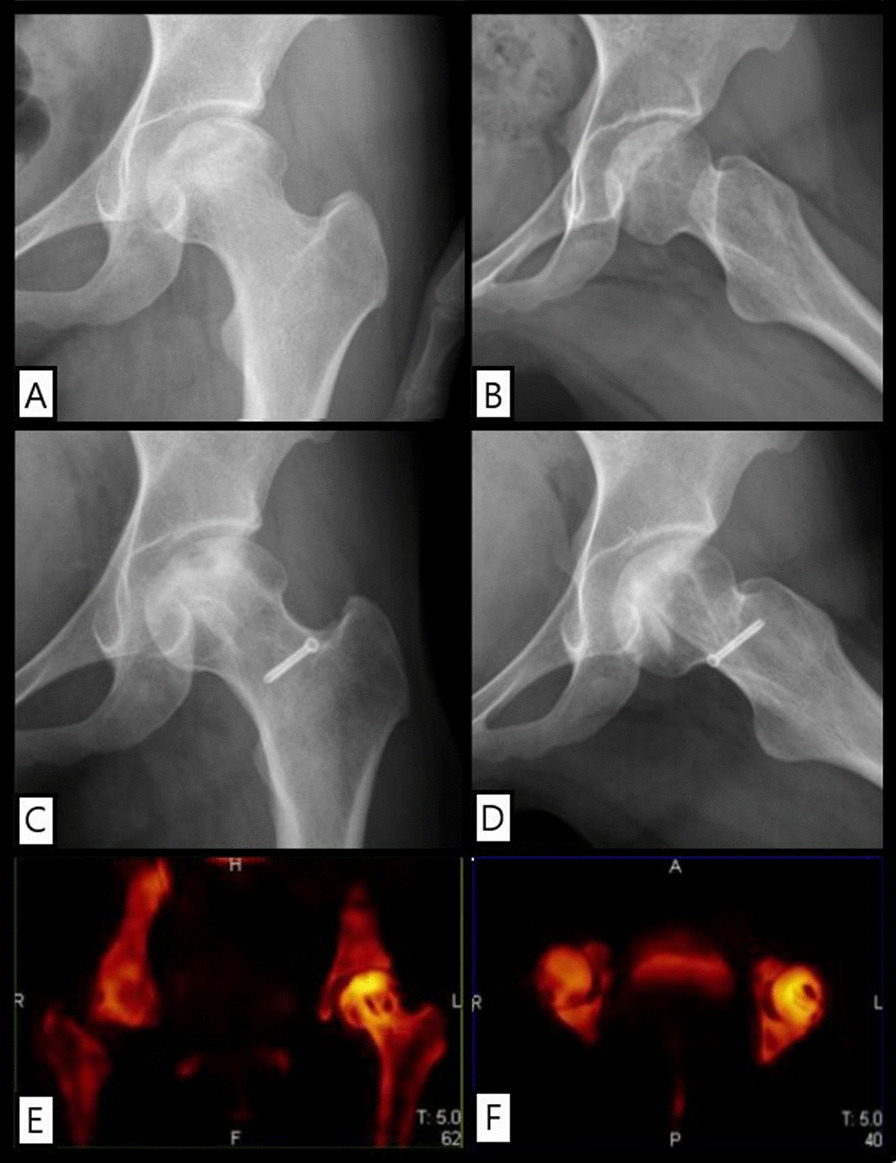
A, B Anteroposterior and lateral hip radiographs; C, D frog position hip radiographs and E, F SPECT/CT of femoral head flow imaging were performed one year after operation
